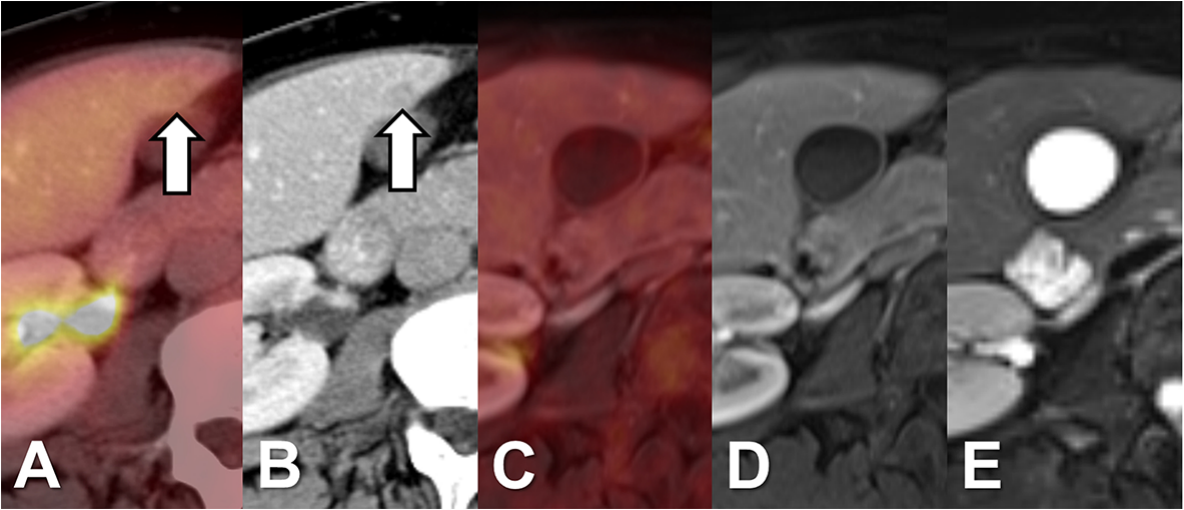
Patient without liver metastases. PET/CT with a false positive result showing a hypodense pseudolesion with a diameter 9 mm in the CT dataset without correlate in PET. In the later acquired PET/MRI (C-E) no correlate in PET nor in the morphological datasets (D: T1w VIBE portal-venous phase; E: T2w TSE fs).
